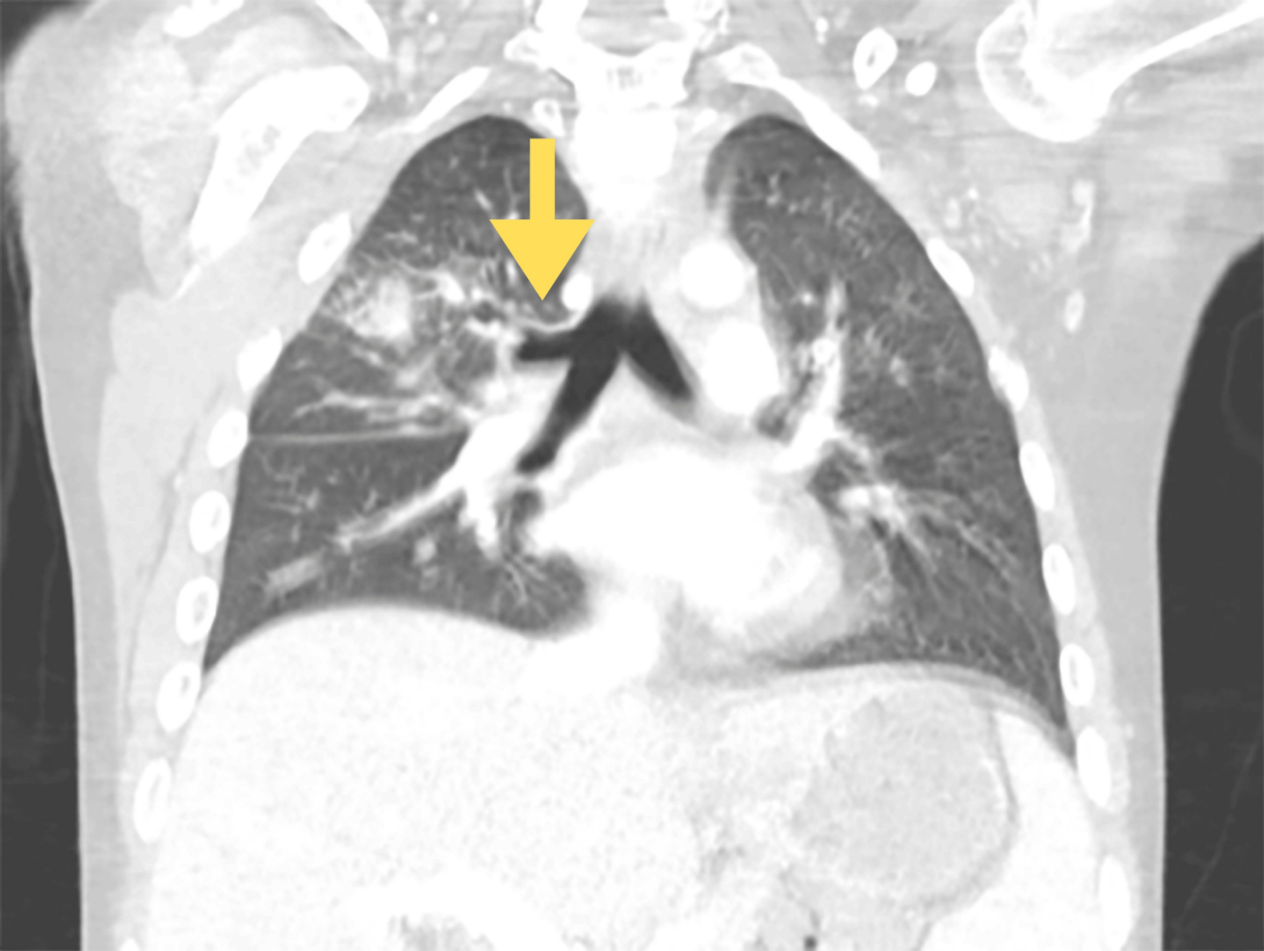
Computed tomography image of right-sided tracheal bronchus
